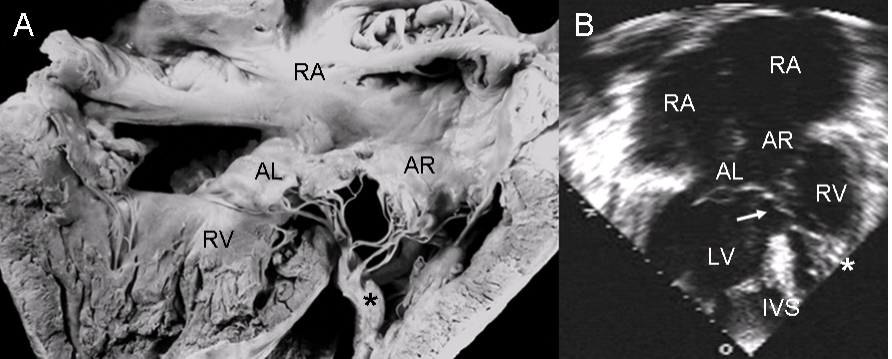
Atrioventricular septal defect with common valve with Rastelli type C insertion. (A) Right heart chambers with insertion of the anterior leaflets in a papillary muscle (asterisk) joined to the right ventricular free wall. (B) Echocardiographic view of four chambers in dextroisomerism shows a similar insertion. Abbreviations as before.
